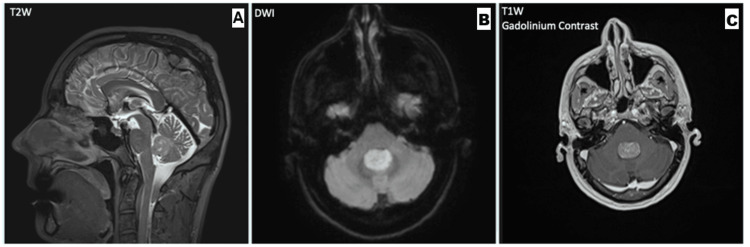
MRI Brain Series (A) Sagittal T2W sequence demonstrating an isointense intraventricular mass without obstructive hydrocephalus. (B) Axial diffusion-weighted MRI demonstrating restricted diffusion (bright). (C) Coronal T1 gadolinium contrast MRI demonstrating homogeneous enhancement. MRI: magnetic resonance imaging; T2W: T2-weighted; DWI: diffusion-weighted imaging; T1W: T1-weighted
